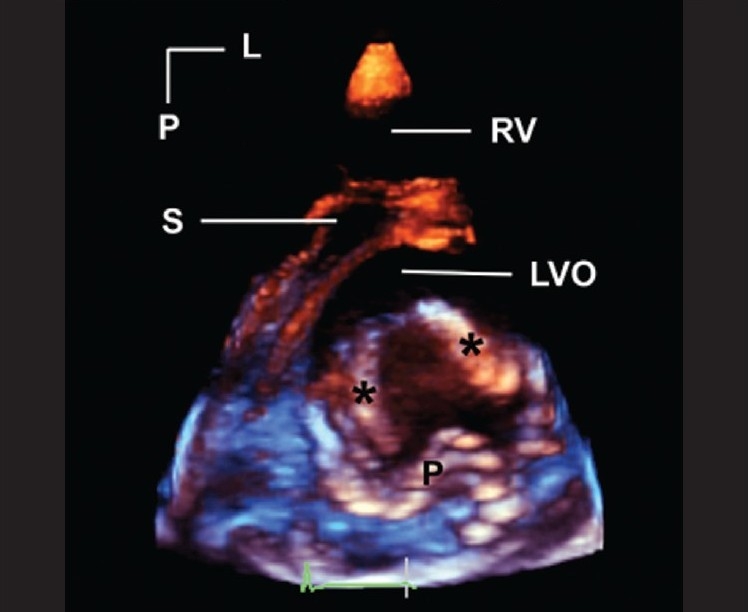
This is a parasternal short axis image of a cleft in the anterior mitral leaflet. Asterisks mark the edges of the cleft. A tissue colorization map has been applied to the image. This has the effect of coloring tissues in the near field (near to either the transducer or the front of the 3D image) orange. Tissues in the far filed are colored blue. This is a dynamic after-effect, which means that as the operator rotates, tilts or otherwise manipulates the image, the color effect correspondingly changes in real-time. L, left; LVO, left ventricular outflow tract; P, posterior; RV, right ventricle; S, septum
